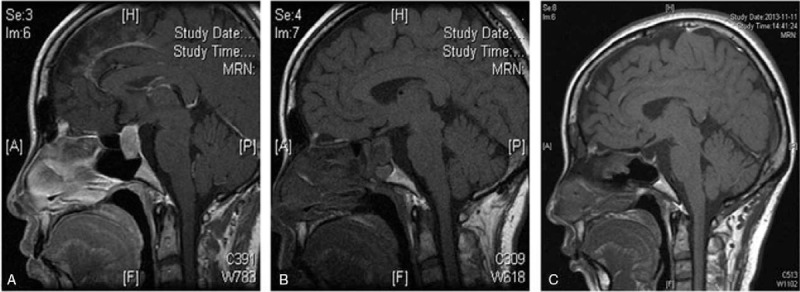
Sagittal magnetic resonance imaging of the head of the female patient in Figure 4. 4.(A) before surgery; (B) 3 months after surgery and before treatment; (C) 47 months after surgery and 44 months after treatment.
